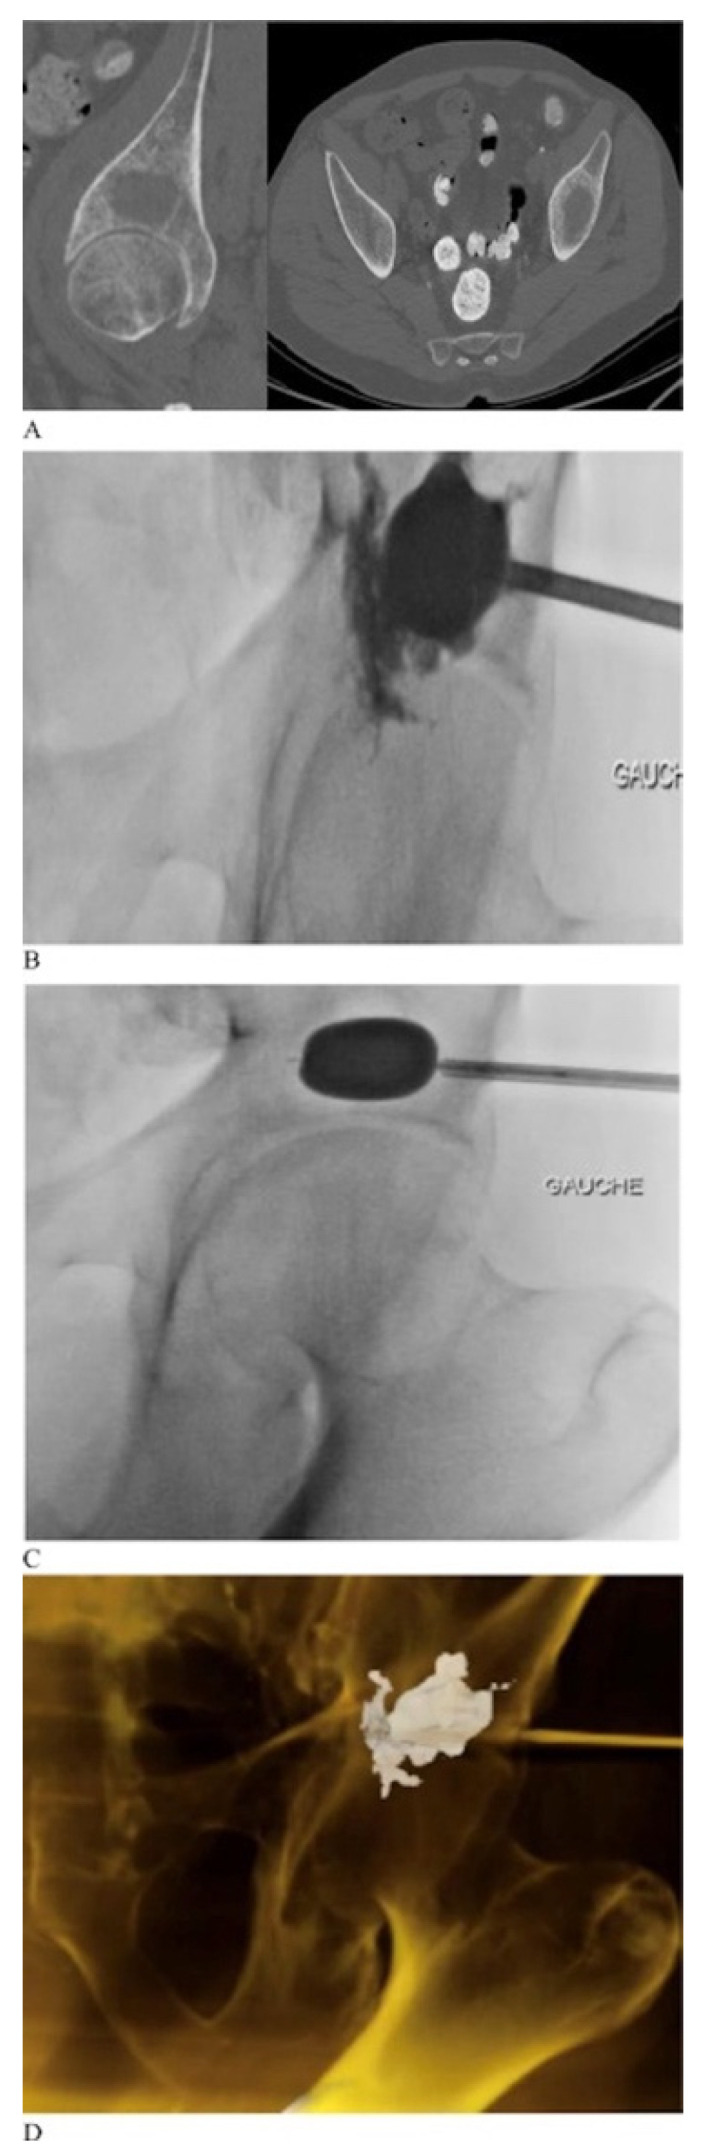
A patient with left superior acetabular metastatic lesion demonstrated on axial and coronal cuts of a preoperative computed tomography scan (A). The patient underwent balloon augmented cementoplasty (B–D). Images borrowed from Couraud et al. (2018) [17].
